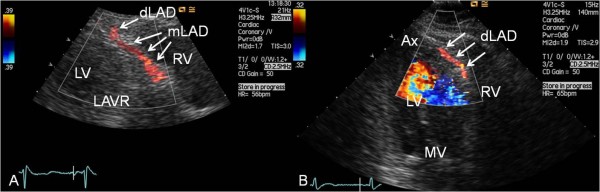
Examples of antegrade coronary artery flow in the middle and distal segments of LAD. (A) The middle left anterior descending coronary artery (mLAD) is imaged from parasternal modified long-axis view focusing on the anterior interventricular sulcus. (B) The distal left anterior descending coronary artery (dLAD) is seen from modified apical 3-chamber view focusing on the anterior interventricular sulcus. Ax = apex of the left ventricle; LAVR = left atrioventricular ring; LV = left ventricle; MV = mitral valve; RV = right ventricle.
